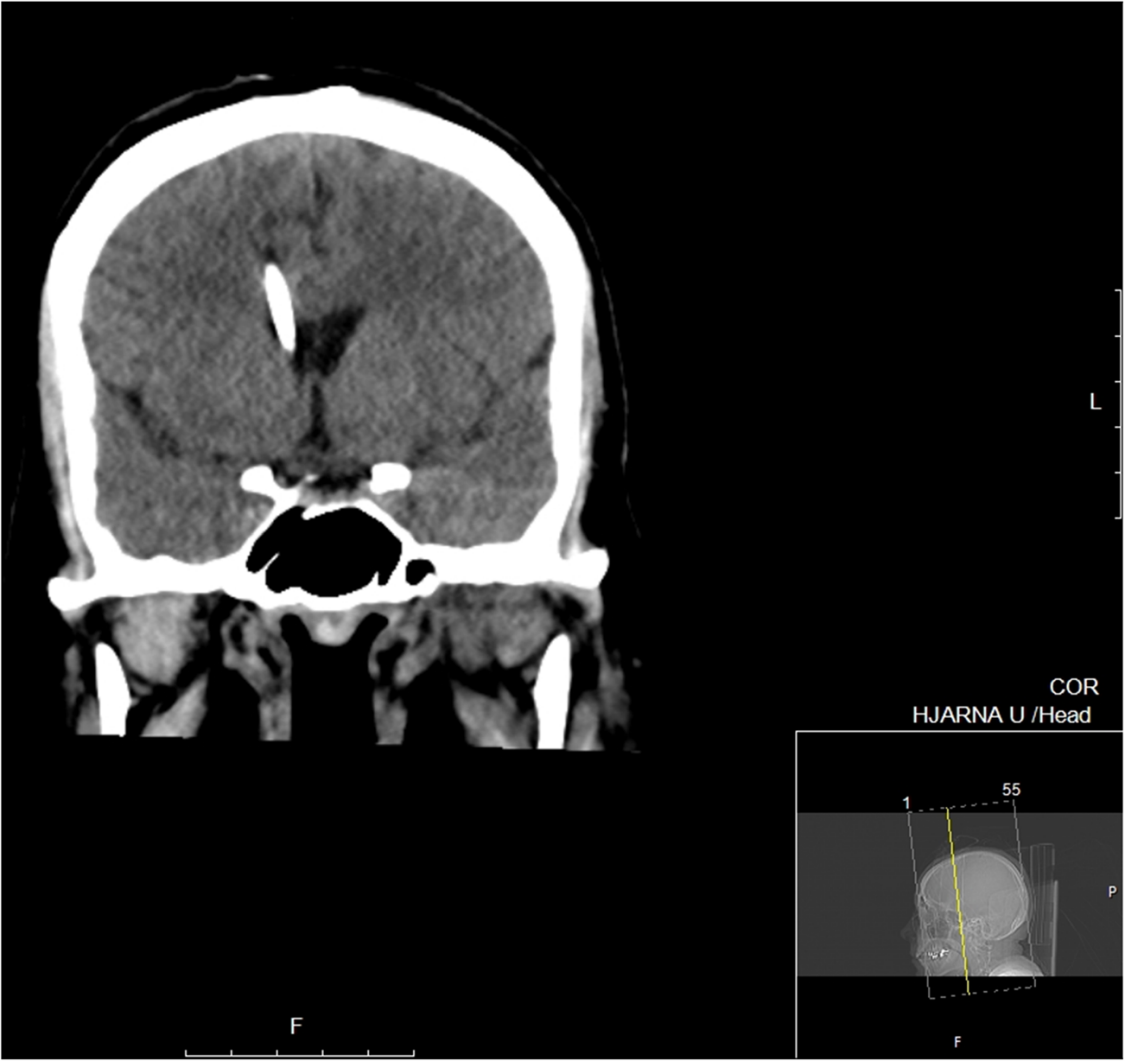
Coronal CT scanning with the EVD in place, with catheter tip at the level of foramen of Monroi (not seen in this projection). Extremely high ICP recordings at 52–60 mmHg was obtained within 30 min of this CT, and the blood pressure was 183/121, resulting in a CPP > 80. Later the same day, a total of 125 mL of CSF was drained through the EVD at an opening pressure of 60 mmHg
